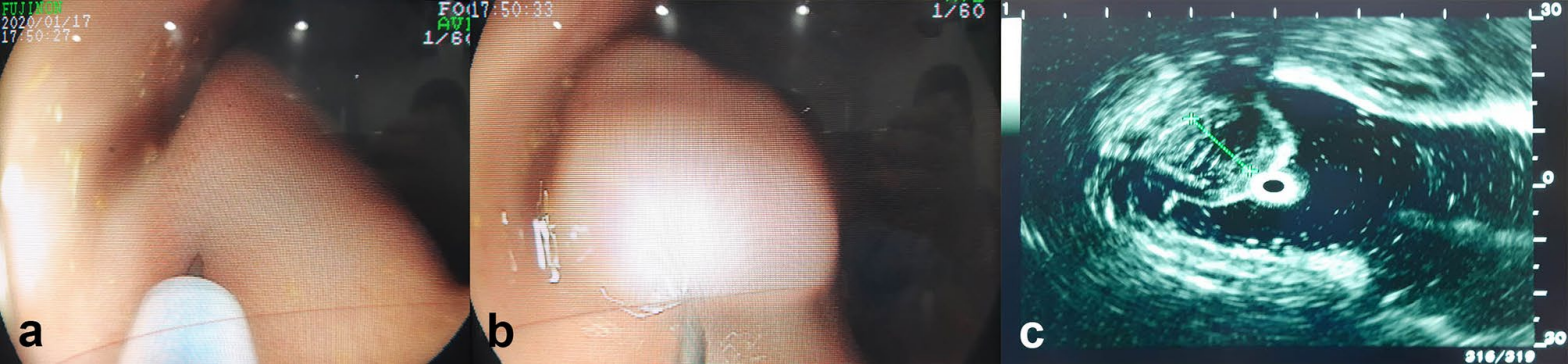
Making submucosal fluid cushions and measuring the height of the cushion by endoscopic ultrasonography. (a) Pre-injection. (b) Post-injection. (c) Ultrasonic view of submucosal fluid cushion.
